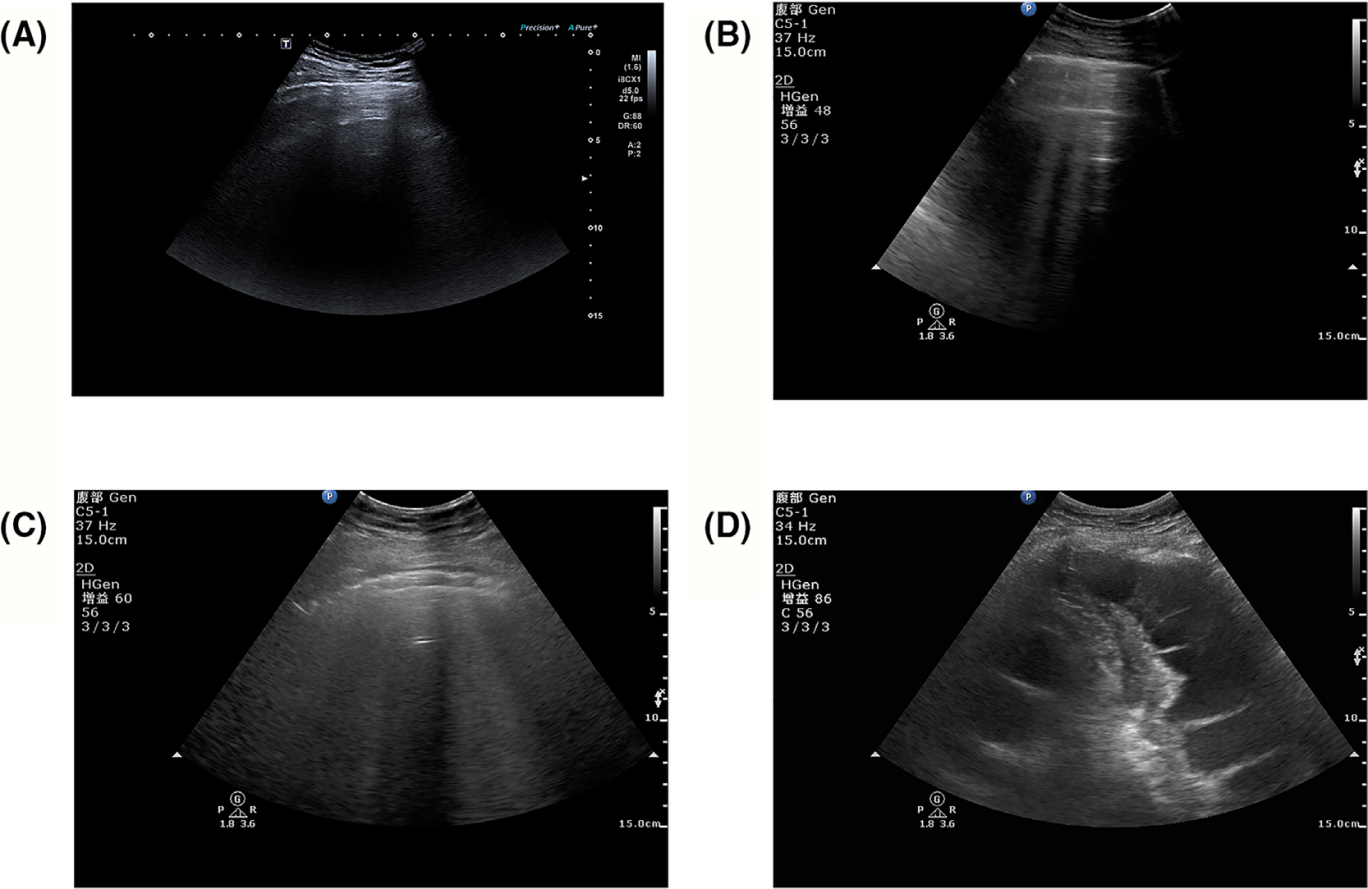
Four types of aeration ultrasound patterns. (A) Presence of A line (scored as 0). (B) Presence of B lines (scored as 1). (C) Presence of coalescent B lines (scored as 2). (D) Presence of lung consolidation (scored as 3).
